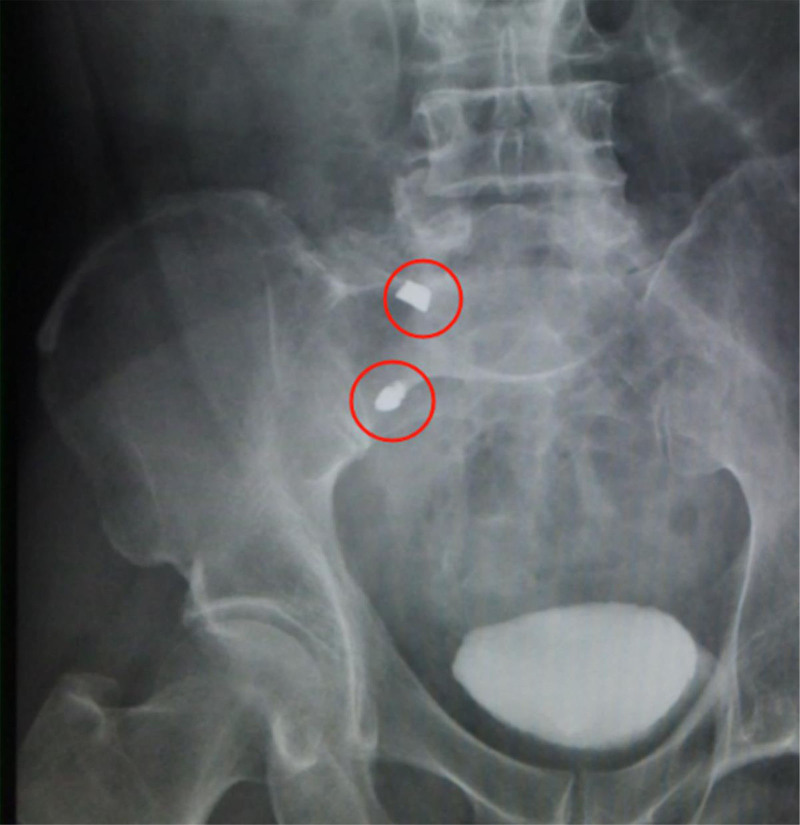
Abdominal X-ray showing 2 metallic objects.
